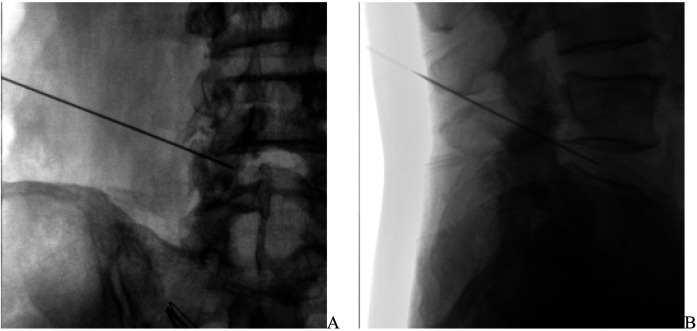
Under the guidance of the C-arm X-ray machine, the puncture needle reaches the target position in the intervertebral disc. (A) The position of the puncture needle on the anteroposterior view. (B) The position of the puncture needle on the lateral view.
